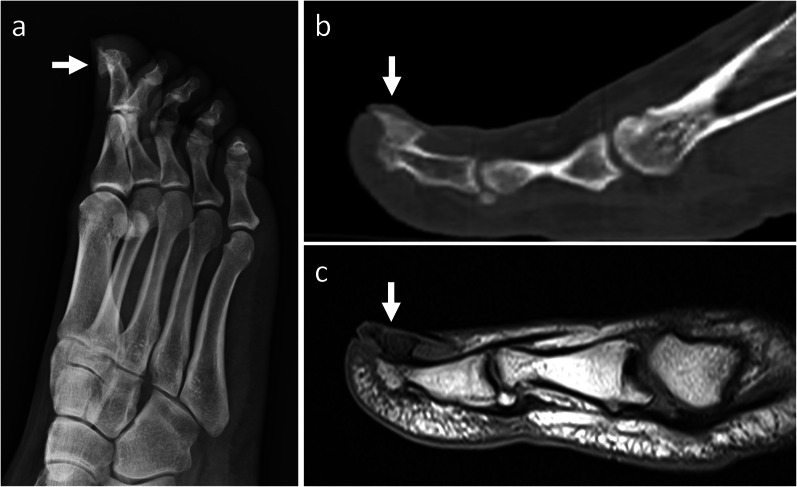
Subungual exostosis. On X-rays (a), a mineralized mass is noted in contiguity with the dorsal aspect of the distal phalanx of the big toe. On sagittal CT (b) and T1-weighted MRI (c) images, no cortical or marrow continuity with the underlying bone is seen. Subungual exostosis was pathologically proven. Arrow points at the lesion in all images
